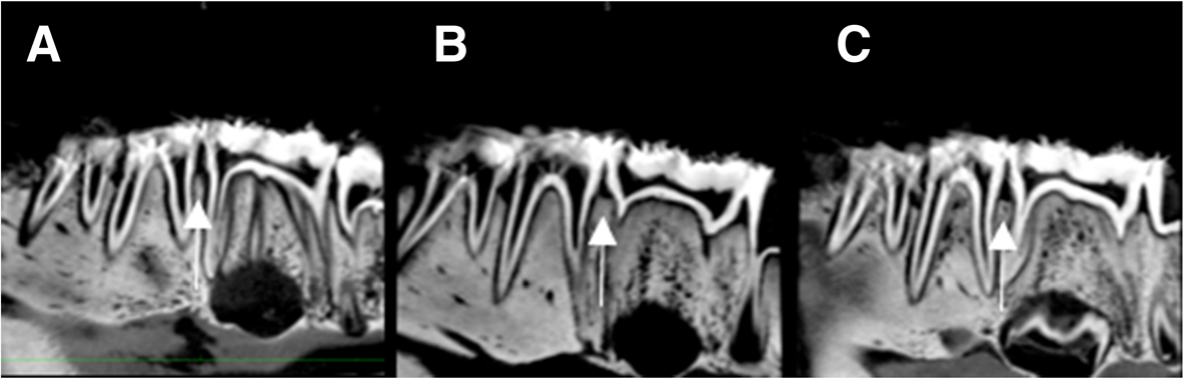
Sagittal sections of CBCT images. a. No lesion (control) between second premolar and first molar. b. Two-hour lesion between second premolar and first molar. c. Four-hour lesion between second premolar and first molar
